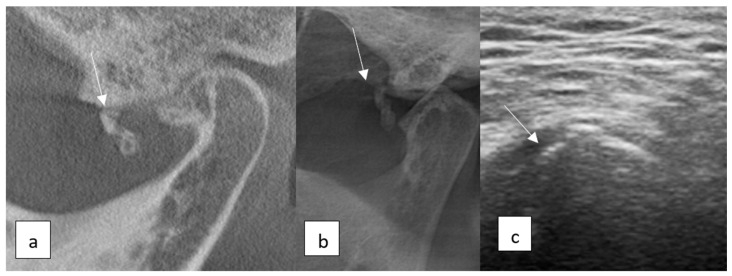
Loose body of TMJ: CBCT image showing loose body (white arrow) and osteophyte on the anterior surface of the condyle (a), panoramic radiography image (b), and USG image (c).
